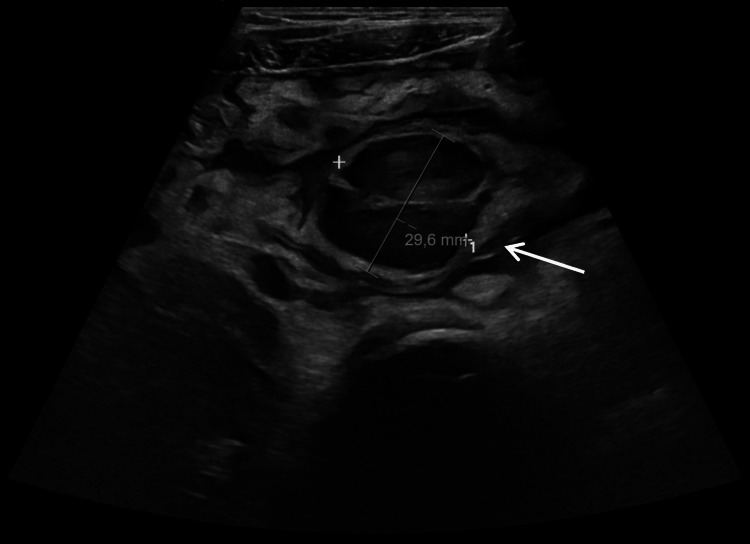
Abdominal ultrasound: hypoechoic nodular structure measuring 30 × 21 mm in the hypogastrium, suggestive of Meckel’s diverticulitis. The arrow indicates the suspected Meckel-related mass.
